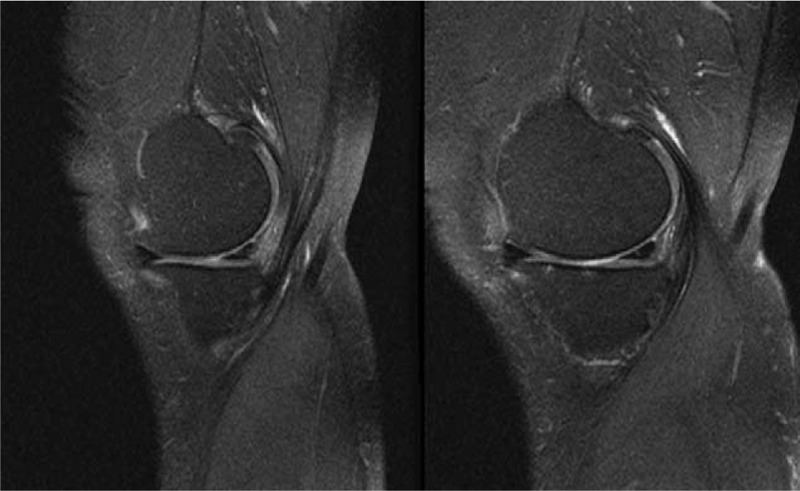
MRI showed degenerative changes in the medial meniscus.
